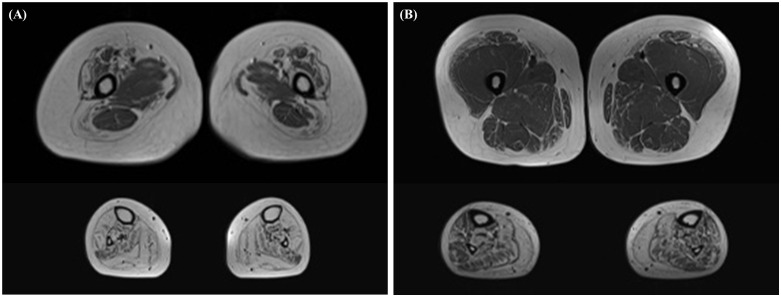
Representative T1-weighted MRI images from proximal-onset and distal-onset ADSS1 myopathy patients. Axial T1-weighted MRI sections at thigh (upper panels) and calf (lower panels) levels from (A) a proximal-onset patient (G-01) and (B) a distal-onset patient (L-01). Both patients show similar patterns of muscle involvement with predominant fatty replacement in distal muscles, particularly the gastrocnemius and soleus, while proximal muscles are relatively less affected.
